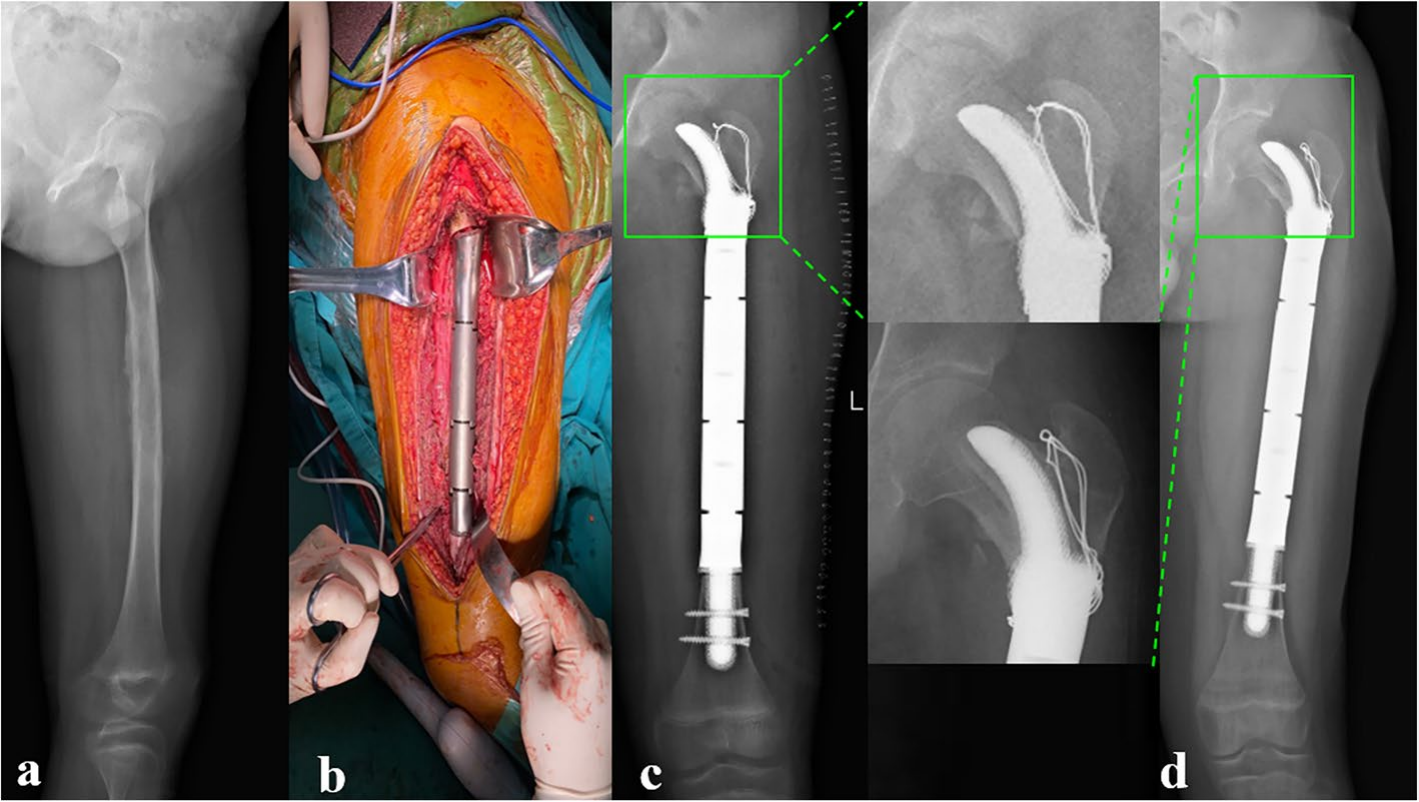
Preoperative and postoperative X-ray evaluations for typical case reconstructed by Stem B: a Preoperative X-ray of femur of Patient 2. b Intercalary femoral replacement was performed. c X-ray of femur taken at 2 days after surgery. d X-ray taken 24 months after surgery. The Stem tip migration occurred (green box)
